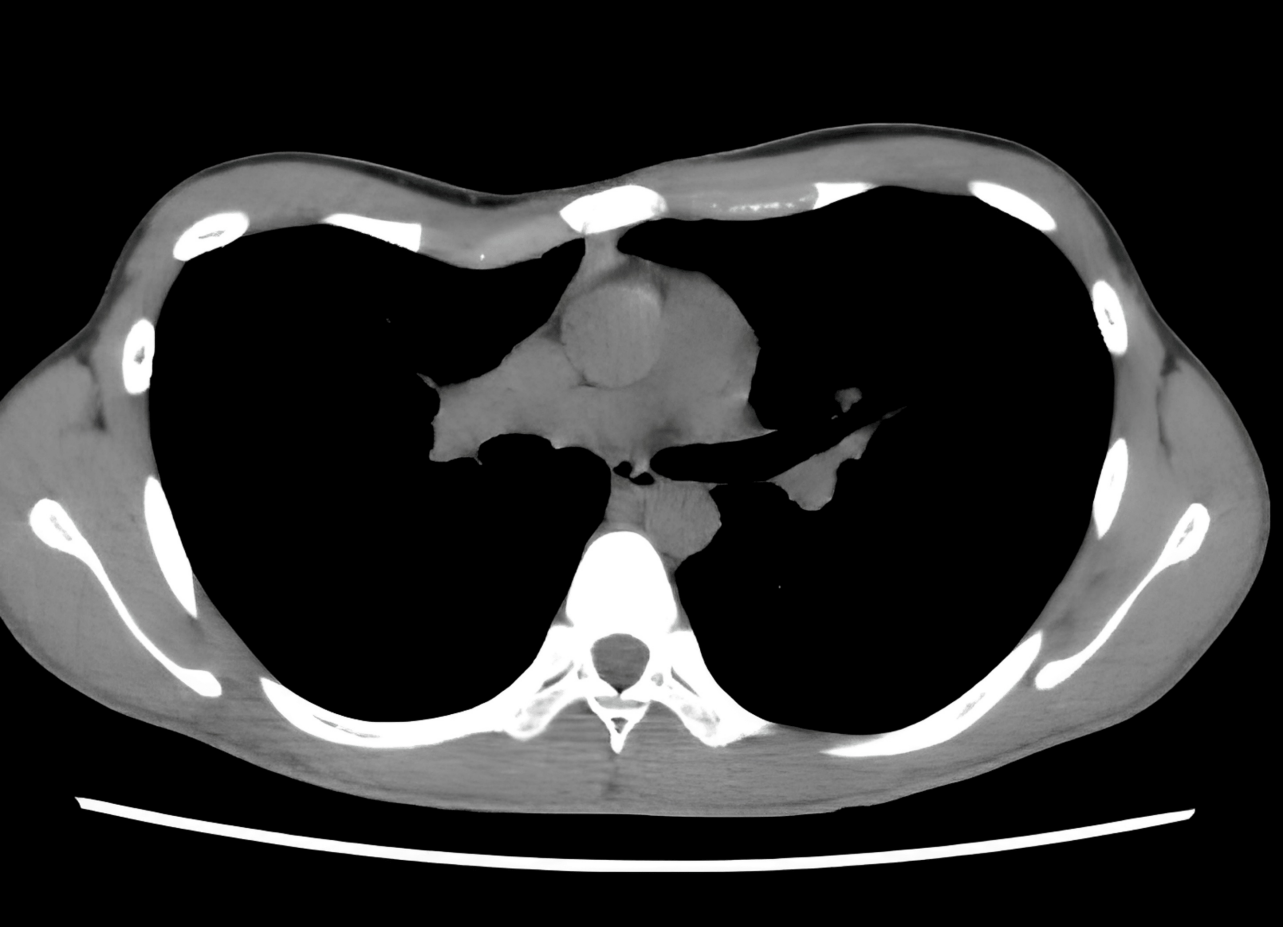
Chest computed tomography (CT) images of Case 1 (Haller index=3.5) Asymmetrical depression is observed in the sternum, predominantly on the right side of the midline.
